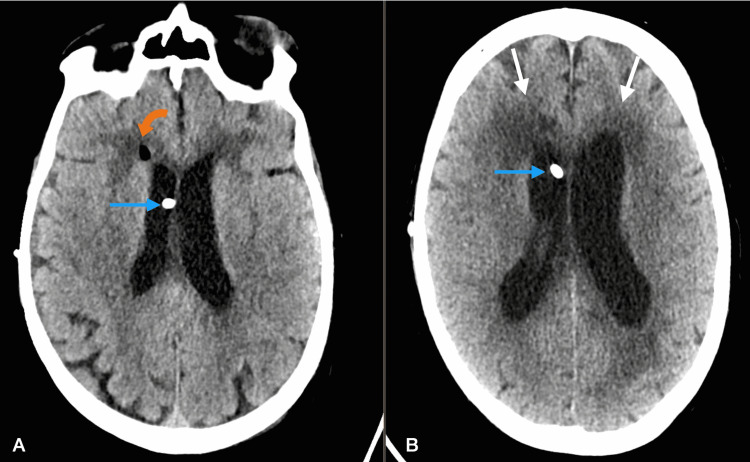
Axial CT image (A) obtained the next day shows almost normal size of ventricles, suggestive of improving hydrocephalus after placement of ventriculostomy catheter, which is seen coursing through the right lateral ventricle (straight blue arrow). A small focus of air (curved orange arrow) is seen, which is a normal postoperative finding. Follow-up axial CT image (B) obtained five days later shows dilatation of the ventricular system and worsened white matter hypoattenuation adjacent to the ventricular system (straight white arrows), suggestive of worsening hydrocephalus despite ventriculostomy catheter placement (straight blue arrow).
